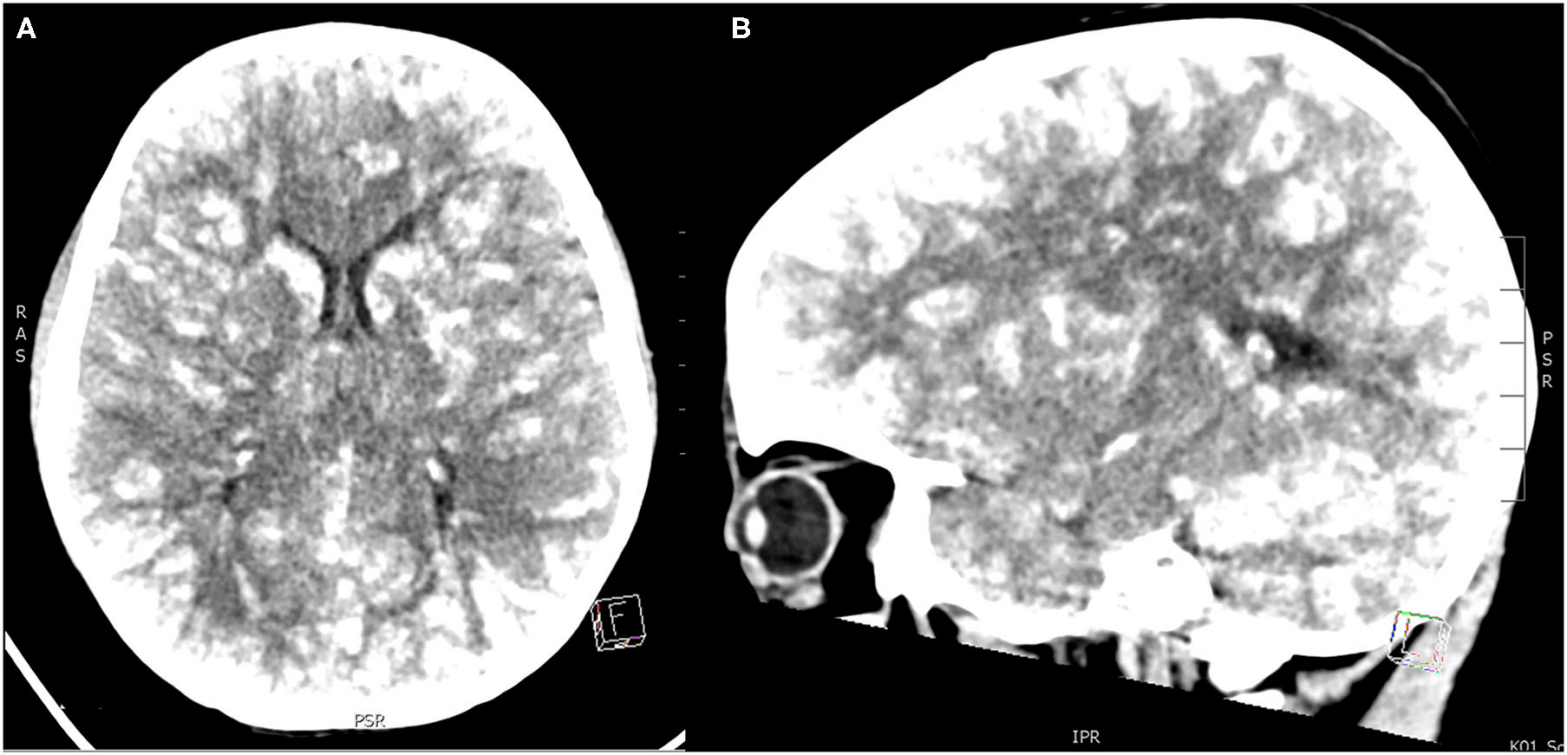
(A,B) Unenhanced head CT images with “lâcher de ballons.” Lipiodol is shown as diffuse high-density lesions in cortical, subcortical region and more profound in the white substance and in cerebral and cerebellar gray cores. (A) axial, (B) sagittal section.
